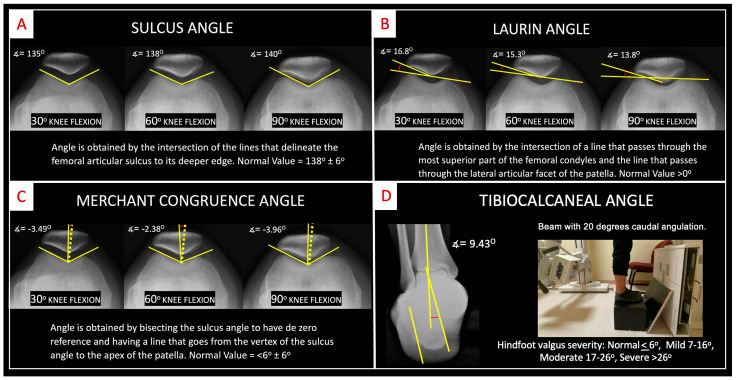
Radiographic evaluation of knee axial projections. Knee axial X-Rays with 30°, 60°, and 90° of flexion and hindfoot X-Ray of the ankle to obtain (A) sulcus angle; (B) Laurin angle; (C) Merchant congruence angle; and (D) the hindfoot alignment X-Ray view with measurement of the tibiocalcaneal angle.
